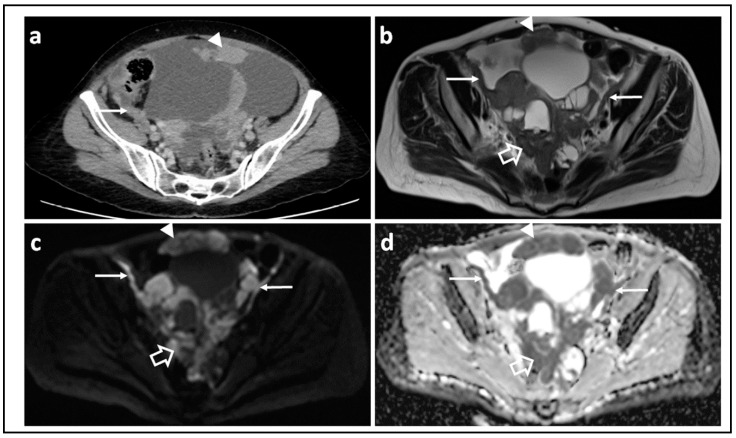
Assessing disease extent using CT versus MRI: CT scan (a) T2-W image (b) and corresponding b = 1050 s/mm^2^ DW-MRI (c) and ADC map (d) through the mid-pelvis in a patient with high grade serous ovarian cancer before treatment. The peritoneal (arrow) and omental disease (arrowhead) is evident in (a), but the extent of peritoneal and serosal disease encasing the sigmoid colon is more striking on MRI (open arrows) where it appears as high signal within the pelvis in (c). Marked diffusion restriction is confirmed in (d).
